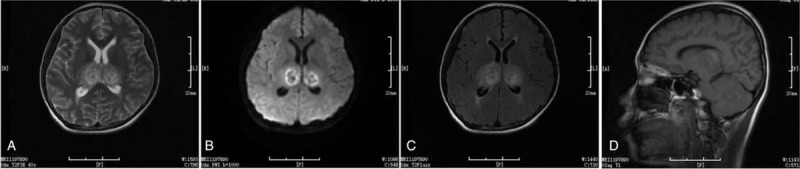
Magnetic resonance imaging of the same patient 7 days after diagnosis with acute necrotizing encephalopathy. The scans showed that lesions of the bilateral dorsal thalamus are slightly reduced, the internal signal obviously uneven, and having short T1 signals. (A) T2. (B) Diffusion-weighted imaging. (C) T2 flair. (D) T1.
